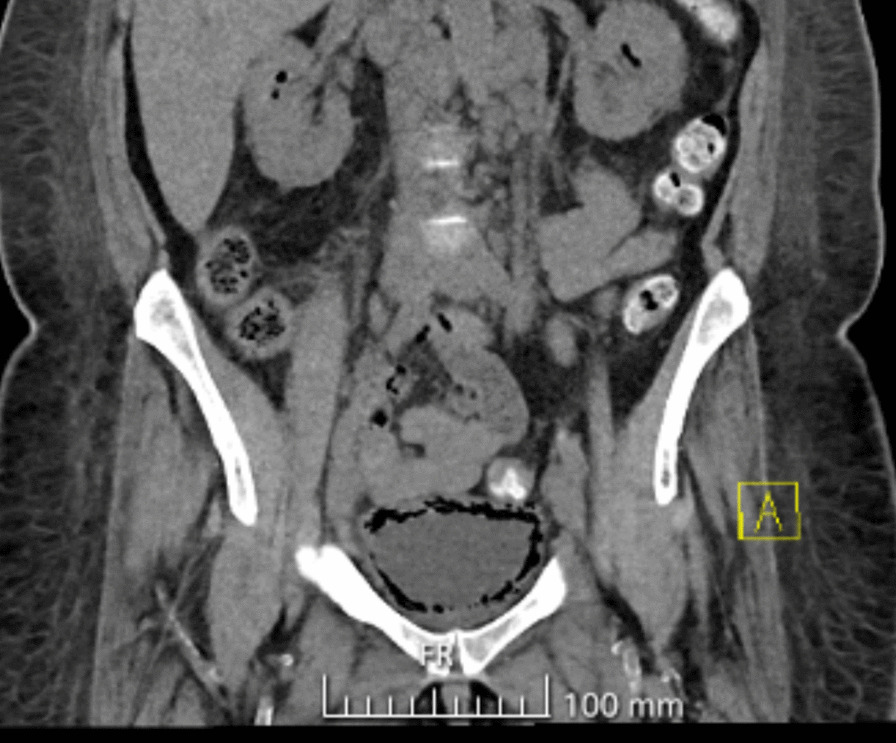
Emphysematous pyelitis and cystitis in the computed tomography of the abdomen
